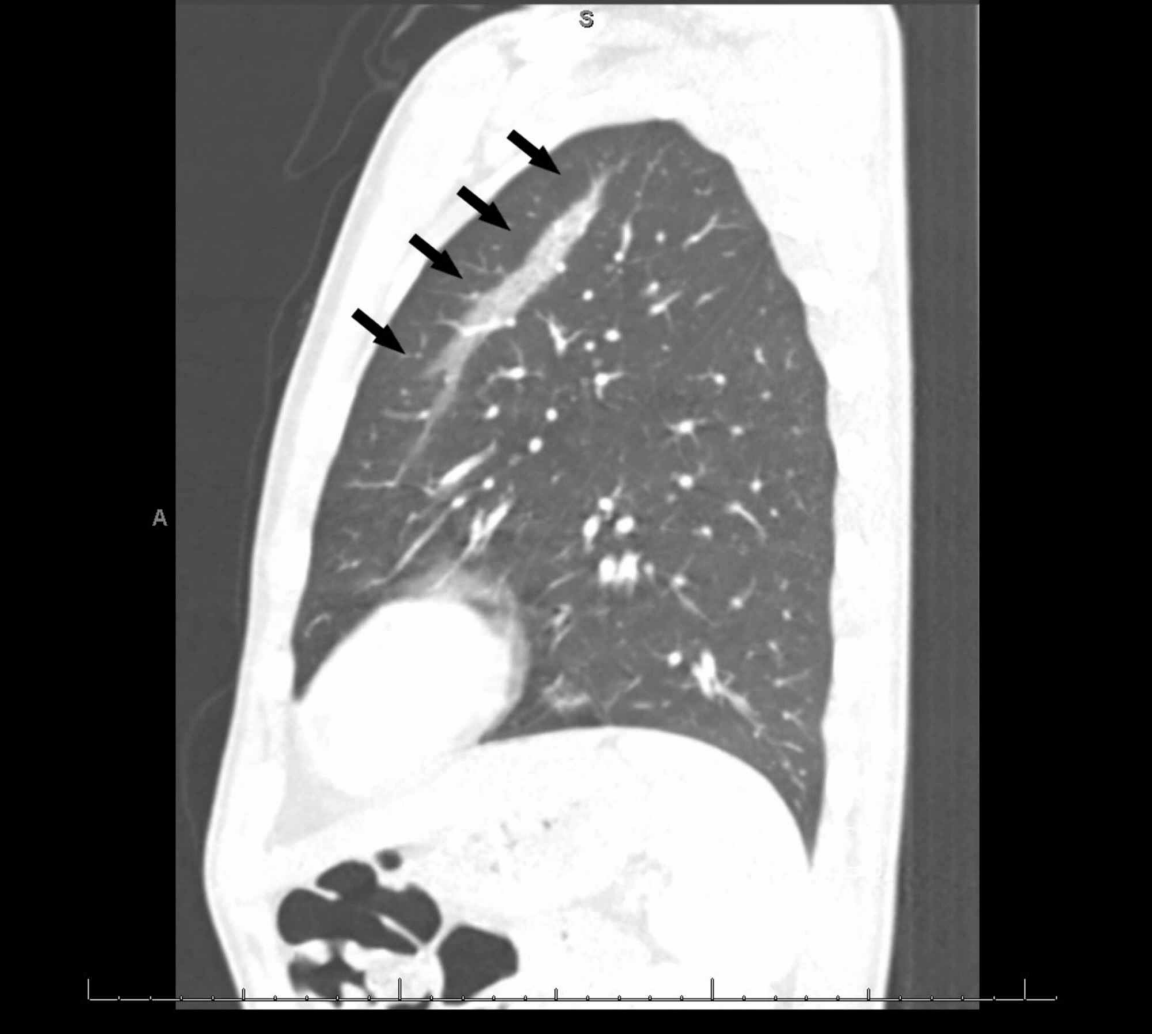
A lateral computed tomography (CT) of the chest revealing changes consistent with ground-glass opacities
